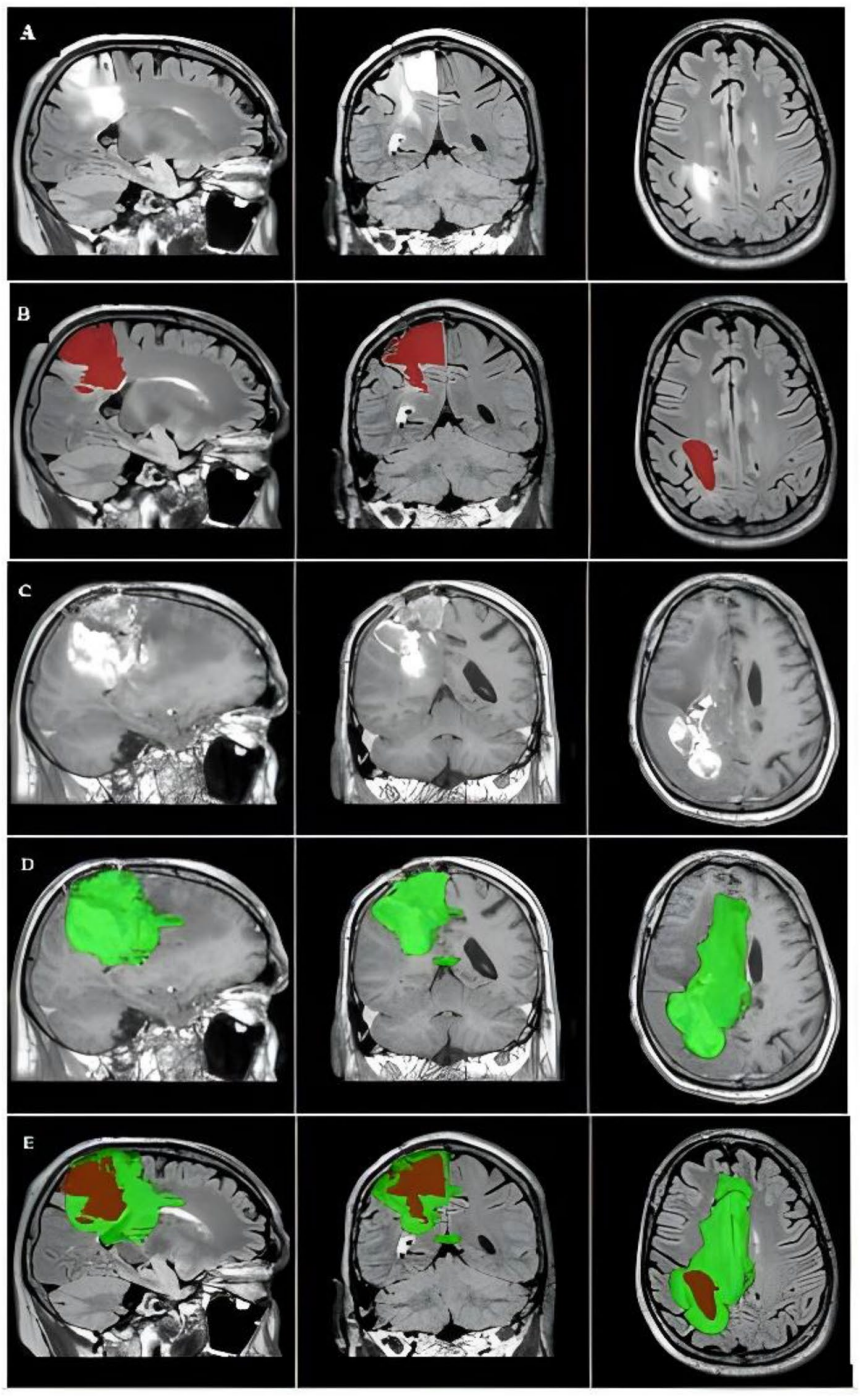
The primary tumor, tumor recurrence, and overlap between the two masks of a representative case. The images were captured in the MNI152 1-mm space. (A) The primary tumor is visualized on preoperative FLAIR image of the right frontal area. (B) Primary tumor mask covers the abnormal region on the preoperative FLAIR image (red). (C) Follow-up CE-T1 shows tumor progression, with the (D) contrast-enhanced tumor recurrence mask on CE-T1 where the tumor recurred (green). (E) Axial plane shows the overlap between the primary tumor (red) and tumor recurrence (green)
